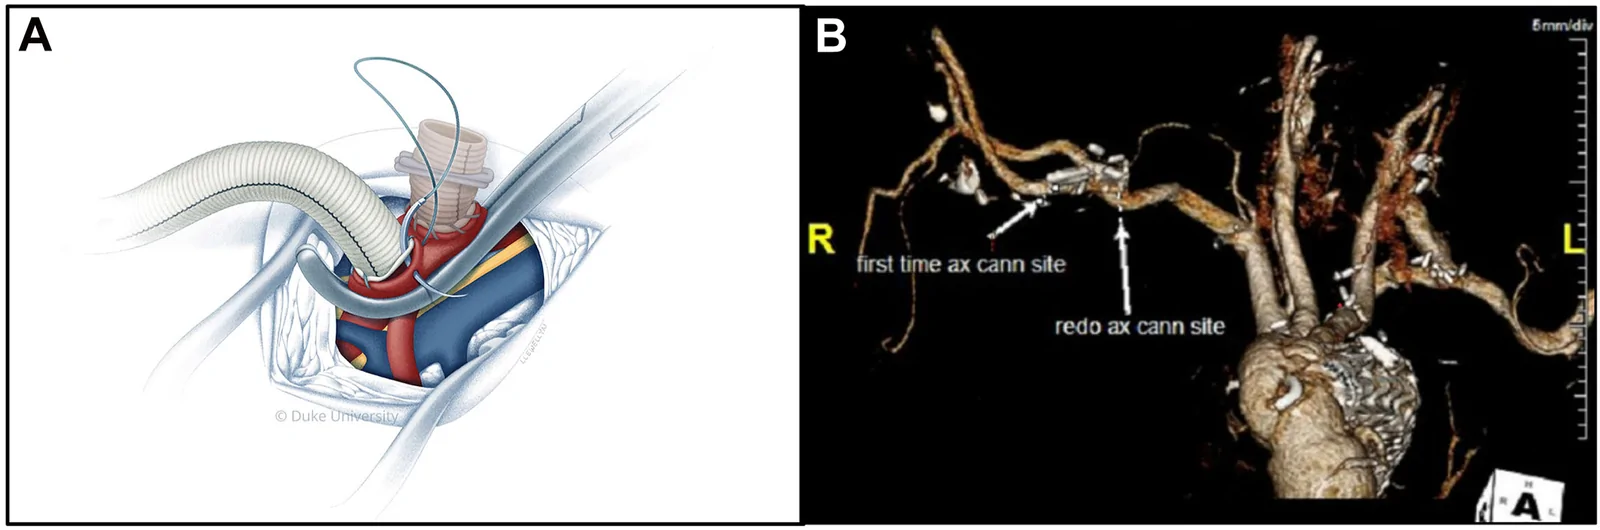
(A) Redo cannulation distal to a prior graft, demonstrating the selection of a new site on the native axillary artery when the original site is less favorable. (B) Postoperative computed tomography angiography 3-dimensional reconstruction image from a patient who underwent redo cannulation proximal to a prior graft, with the original and redo sites along the artery indicated. Note the visible clips where the prior graft stumps were clipped and oversewn. (ax cann, axillary cannulation; L, left; R, right.)
